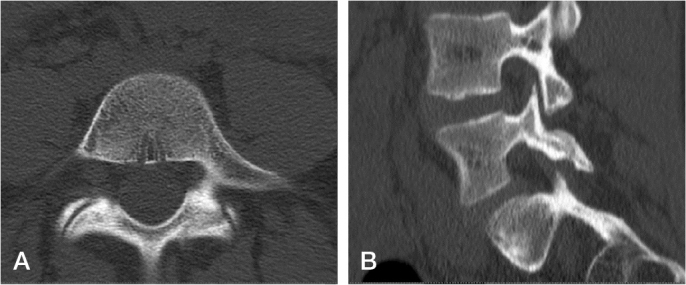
Radiographic healing of the progressive unilateral spondylolysis on CT after 4 months’ activity restriction. (A) Axial and (B) sagittal reformatting demonstrate bony bridging of the stress fracture, although trabeculation is not complete.
